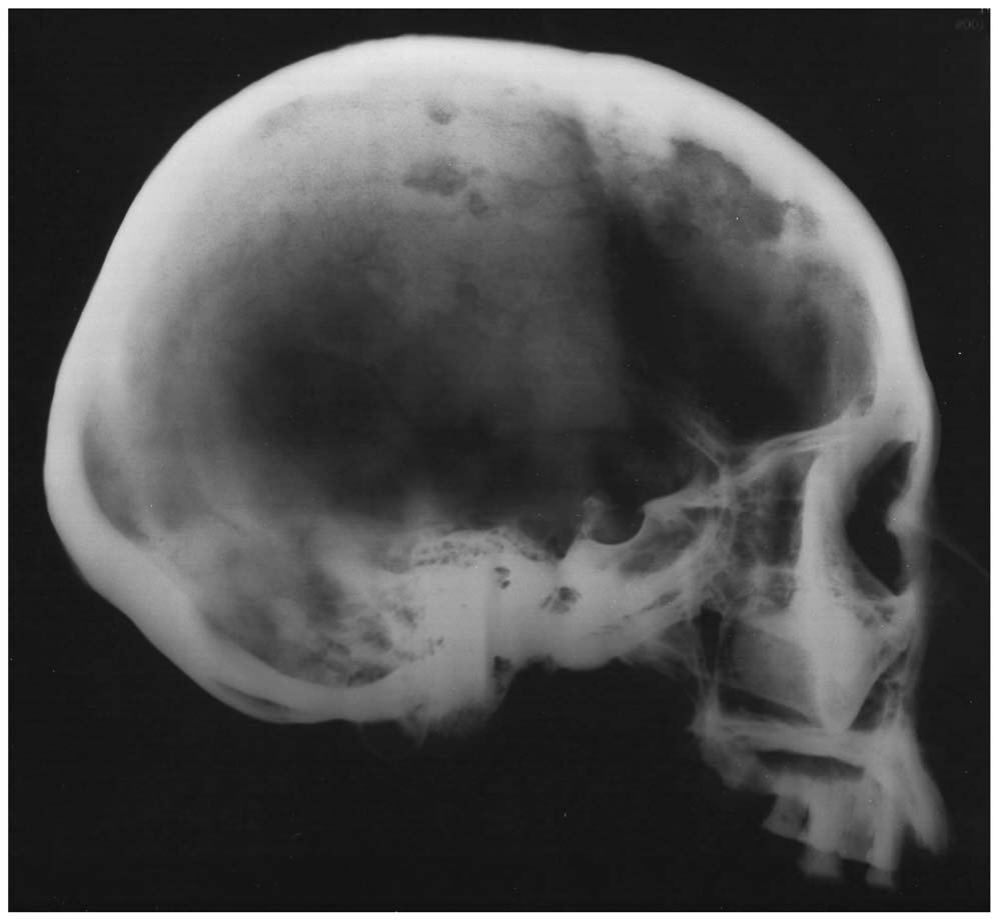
Lateral radiograph of the cranium with at least three areas of diplöic rarification (reduced radio-opacity) on the parietal bones, indicative of additional lesions.
